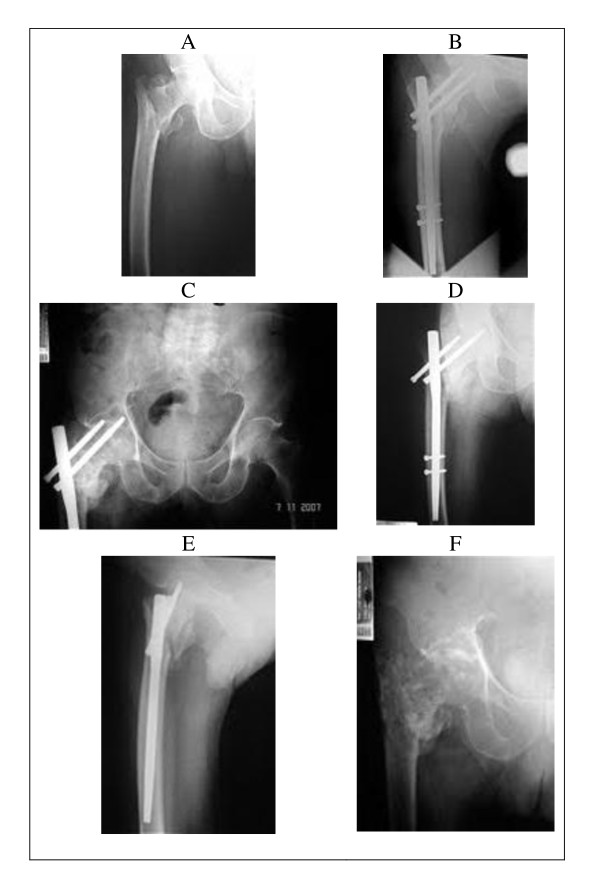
Case 2 - Radiographic images showing the intertrochanteric fracture that was fixed with a proximal femoral nail - (A) preoperative and (B) postoperative; (C and D) the lateral migration of the superior screw and perforation of the femoral head by the inferior screw (reverse Z-effect); (E) the incorrect positioning of the interlocking screws in the lateral incidence, together with poor bone material, may have been responsible for the reverse Z-effect; (F) the removal of the material of osteosynthesis is shown together with signs of chondrolysis of the hip.
